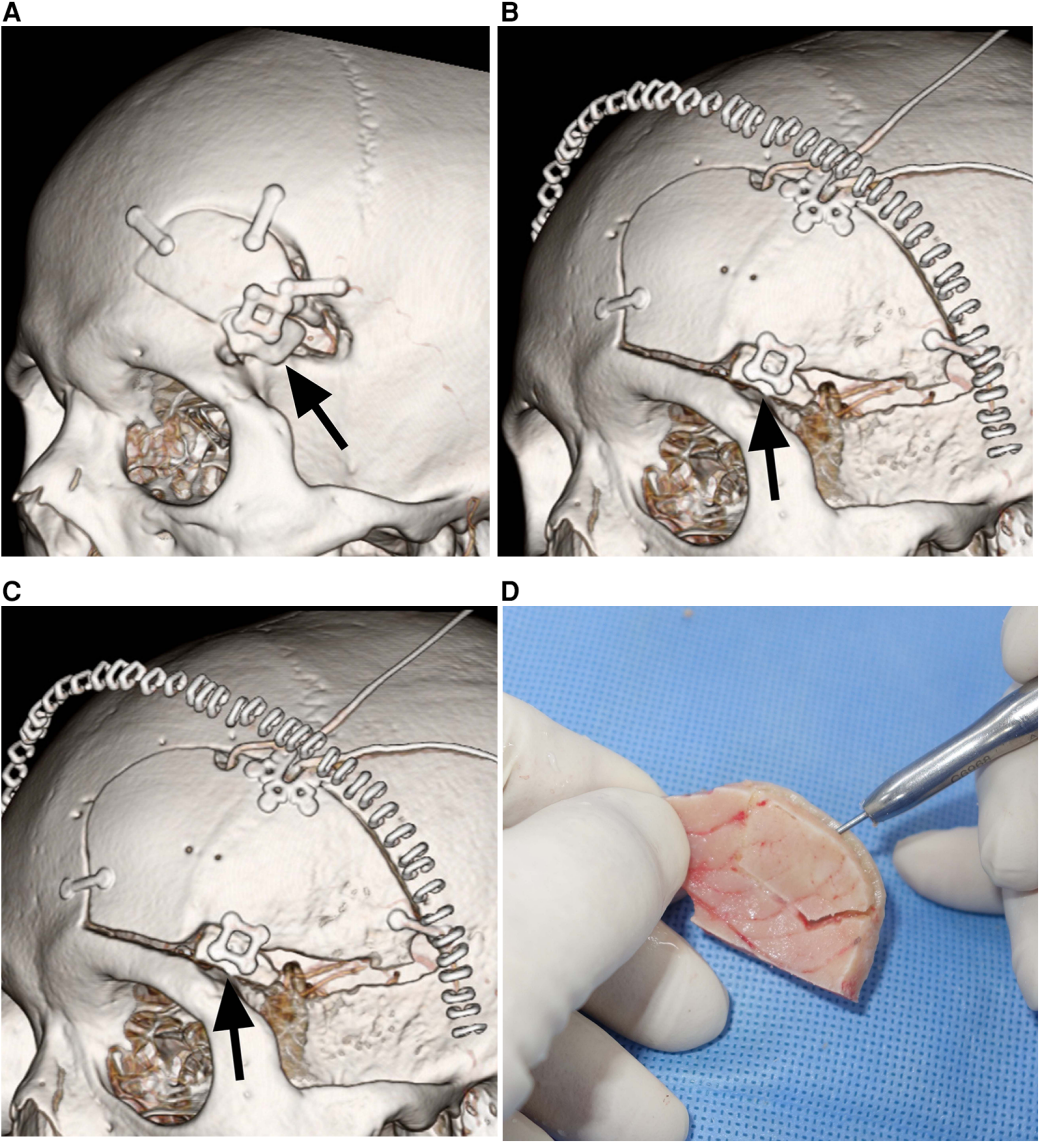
Durability of split inner table grafts. (A) Postoperative CT showing a mini-pterional craniotomy bone flap and the split inner table graft (*arrow*) for reconstruction in Patient 5. (B) CT 12 months after the craniotomy and reconstruction demonstrating the excellent durability of the split inner table graft (*arrow*) in Patient 5. (C) Postoperative CT showing a conventional pterional craniotomy bone flap and the split inner table graft (*arrow*) for reconstruction in Patient 7. (D) CT 12 months after the craniotomy and reconstruction showing 26.9% resorption of the split inner table graft (*arrow*) in Patient 7. The bone resorption is mainly limited to the posterior part of the inner table graft that is covered by the temporalis muscle.
